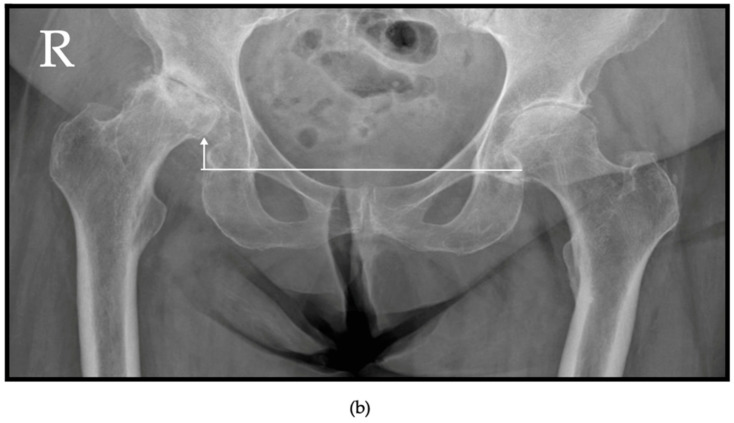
Right RPOH in a 68-year-old female patient—AP views of the pelvis, horizontal white line—connecting the pelvic tear drops; (a) subchondral insufficiency fracture located at the weight-bearing area of the right femoral head (magnified area—red arrow) with superior joint space narrowing and no ascension of the femoral head; (b) femoral head and acetabular bone resorption with significant ascension of the femoral head. The patient presented with pain during walking in August 2022 (a) with a pelvic AP view demonstrating Zazgyva Grade I RPOH; in August 2023 (b), a pelvic AP radiographic view demonstrated destruction of the femoral head accompanied by acetabular involvement with elevation of the femoral head exceeding 0.5 cm, corresponding to Zazgyva Grade III, white arrow—ascension of the femoral head from the tear drop line.
